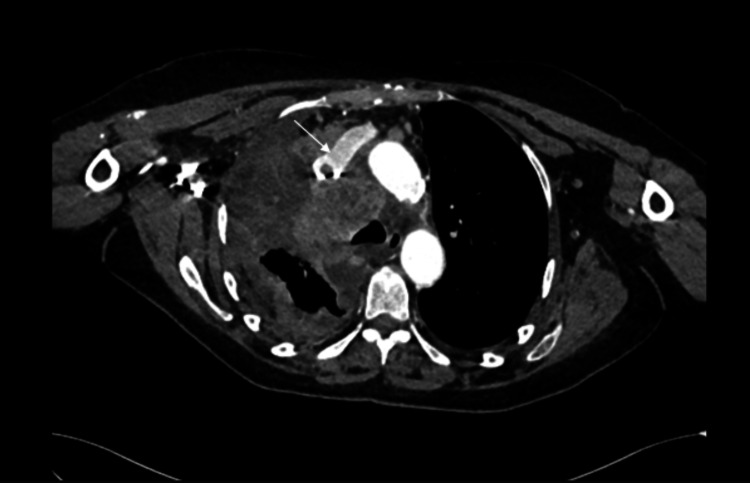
CT thorax showing a narrowed superior vena cava with tumor infiltration (white arrow).
